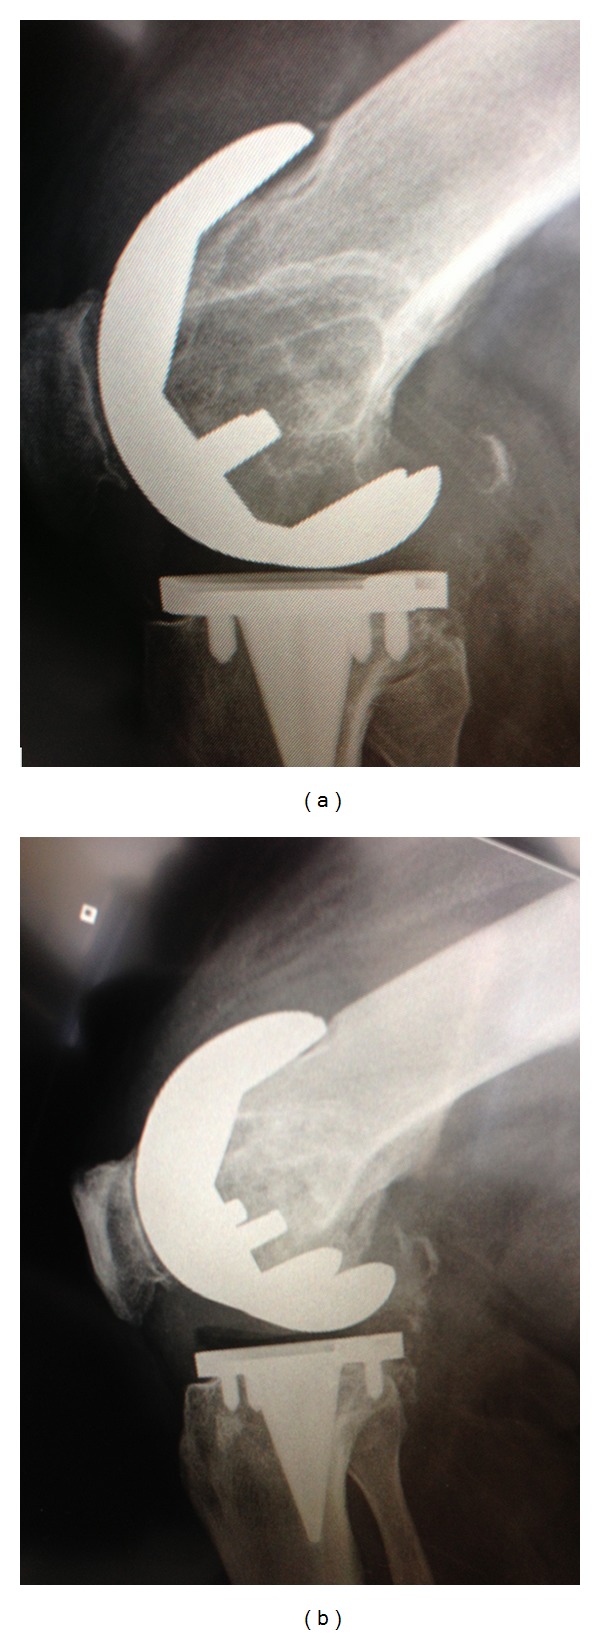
Radiographs of a 67-year-old male who underwent bone grafting and isolated insert exchange for femoral osteolytic region. (a) The prerevision radiograph. (b) Three-month postrevision radiograph.
